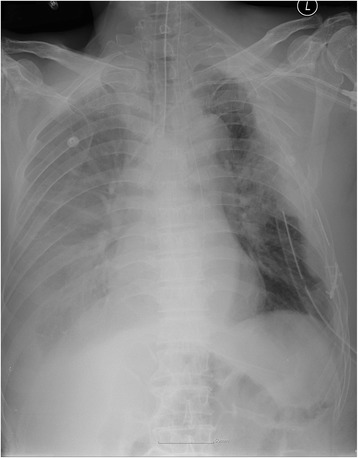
Chest X-ray on fifth postoperative day after open reduction of multiple ribs fracture on left side
